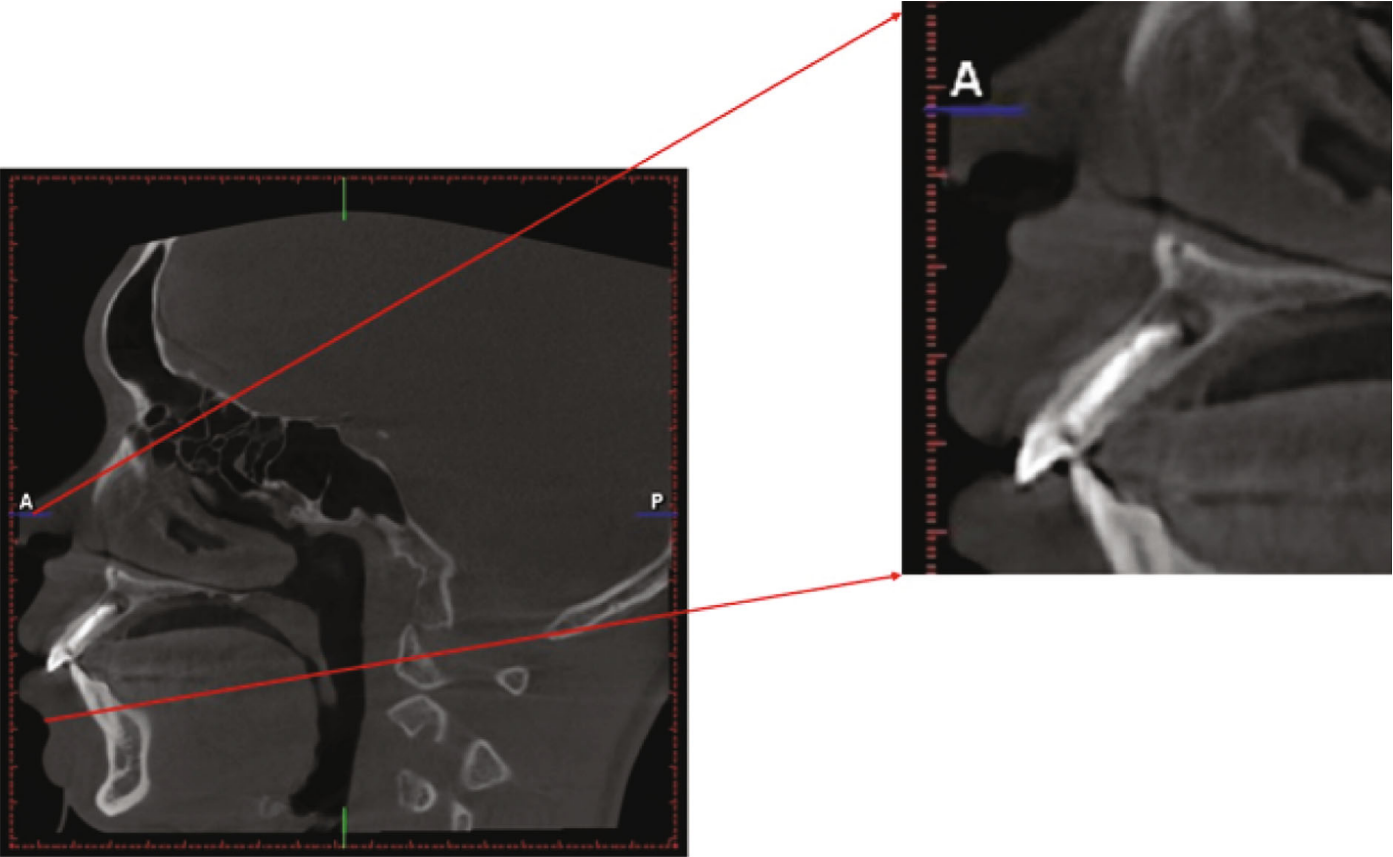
Cone Beam Computed Tomography (CBCT) of tooth 22 with a periapical lesion.
